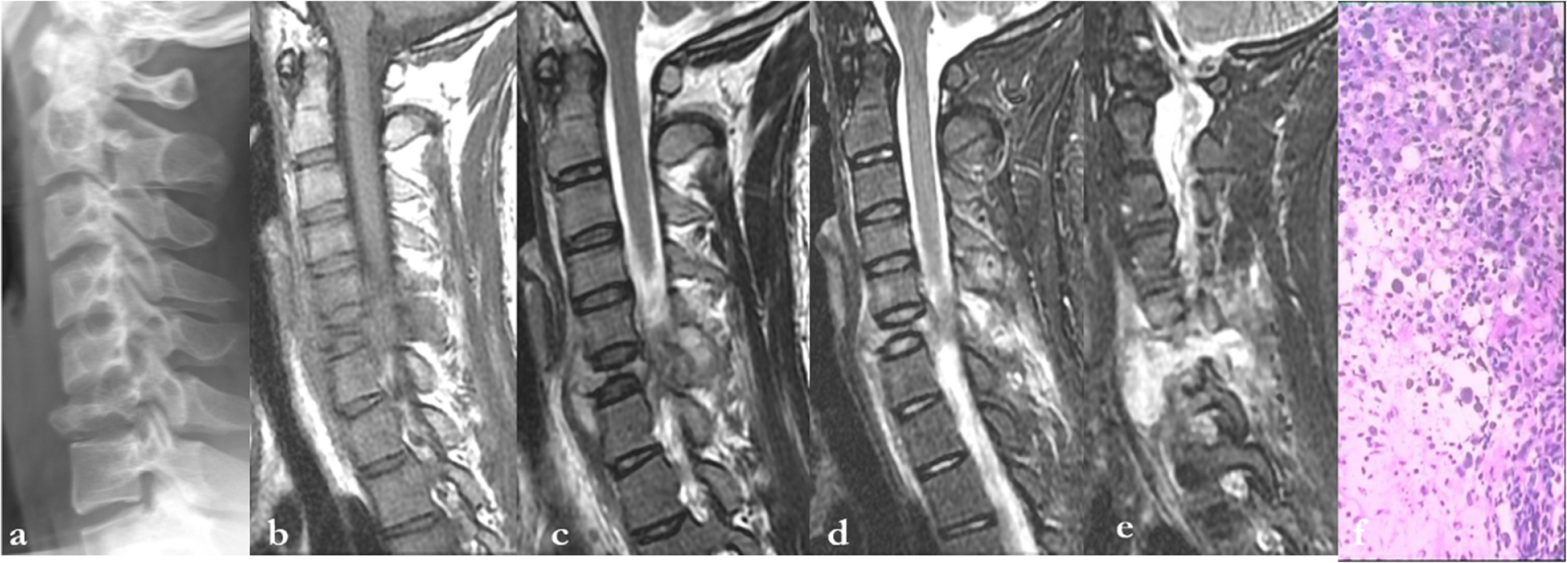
Spinal X-ray and sagittal MR image of the cervical spine of a 19-year-old male patient with neck pain and restricted neck motion. X-ray (a) shows a lesion in the C6 associated with severe vertebral collapse. The lesion shows slight hypointensity on T1WI (b), hyperintensity on T2WI (c), and hyperintensity on fat-saturated T2WI (d, e), associated with obvious marrow and soft tissue edema, and prevertebral and epidural soft tissue extension. The diagnosis of LCH was confirmed by pathology after surgery (pathological slice HE × 200) (f)
